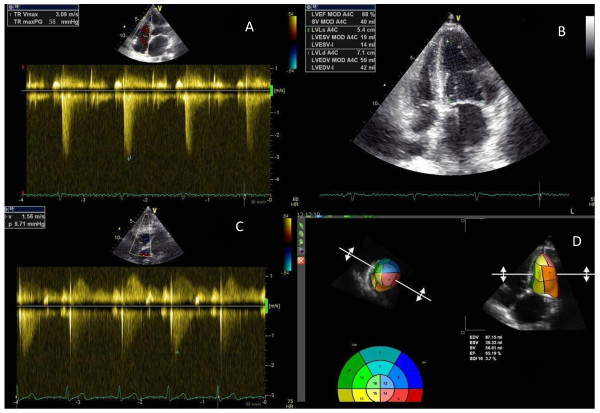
Transthoracic cardiac ecocolordoppler showing evidence of pulmonary hypertension (A-B) and normal pulmonary hypertension and ejection fraction (C-D).
